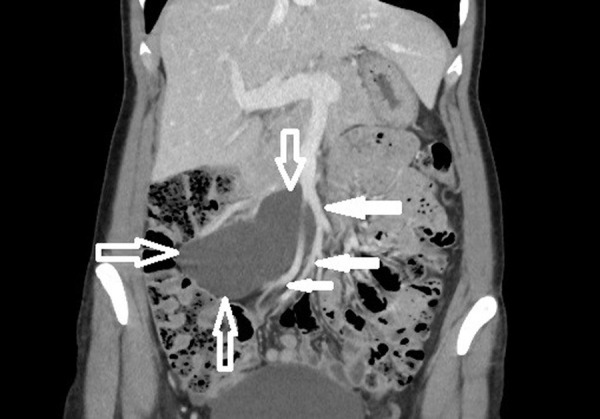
CT scan of the lymphangioma (open arrows) in right abdomen area. The mass extends from pancreatic head and superior mesenteric vein near ileum to right iliac area along the ileocolic artery and vein. Superior mesenteric vein and ileal veins are visible (bold arrows).
